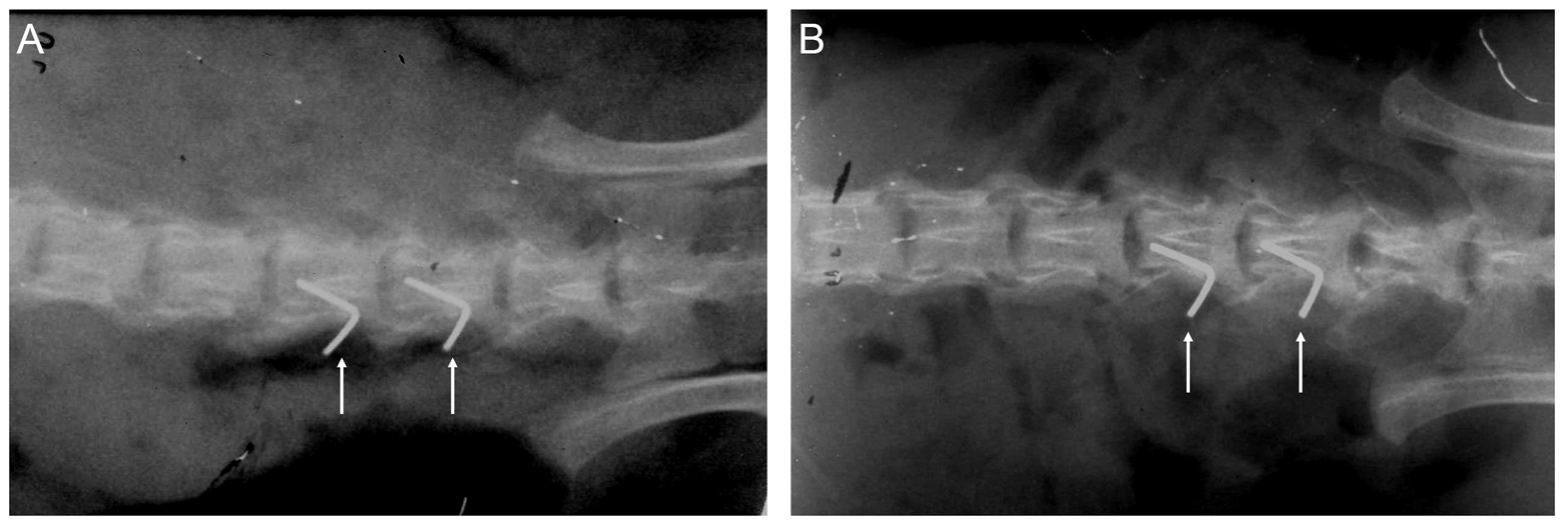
A soft X-ray radiographic analysis of CCD, both immediately following CCD surgery (A) and 8 days post-surgery (B); the arrows indicate the position of the L-shaped needles.
